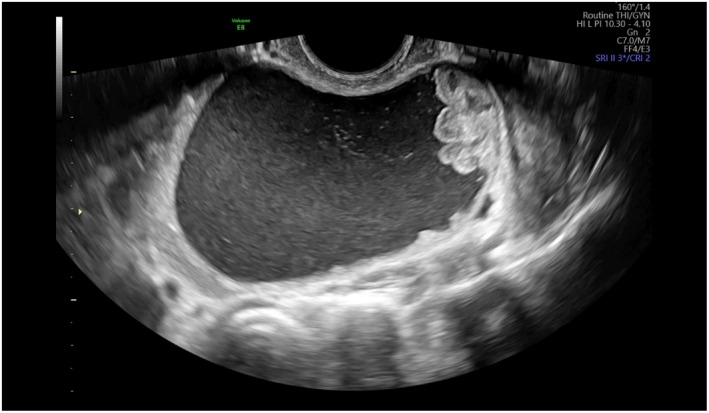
Transvaginal gray scale ultrasound image showing an axial view of a seromucinous borderline ovarian tumor, that appears as an unilocular solid cyst with ground‐glass content and one papillary projection.
